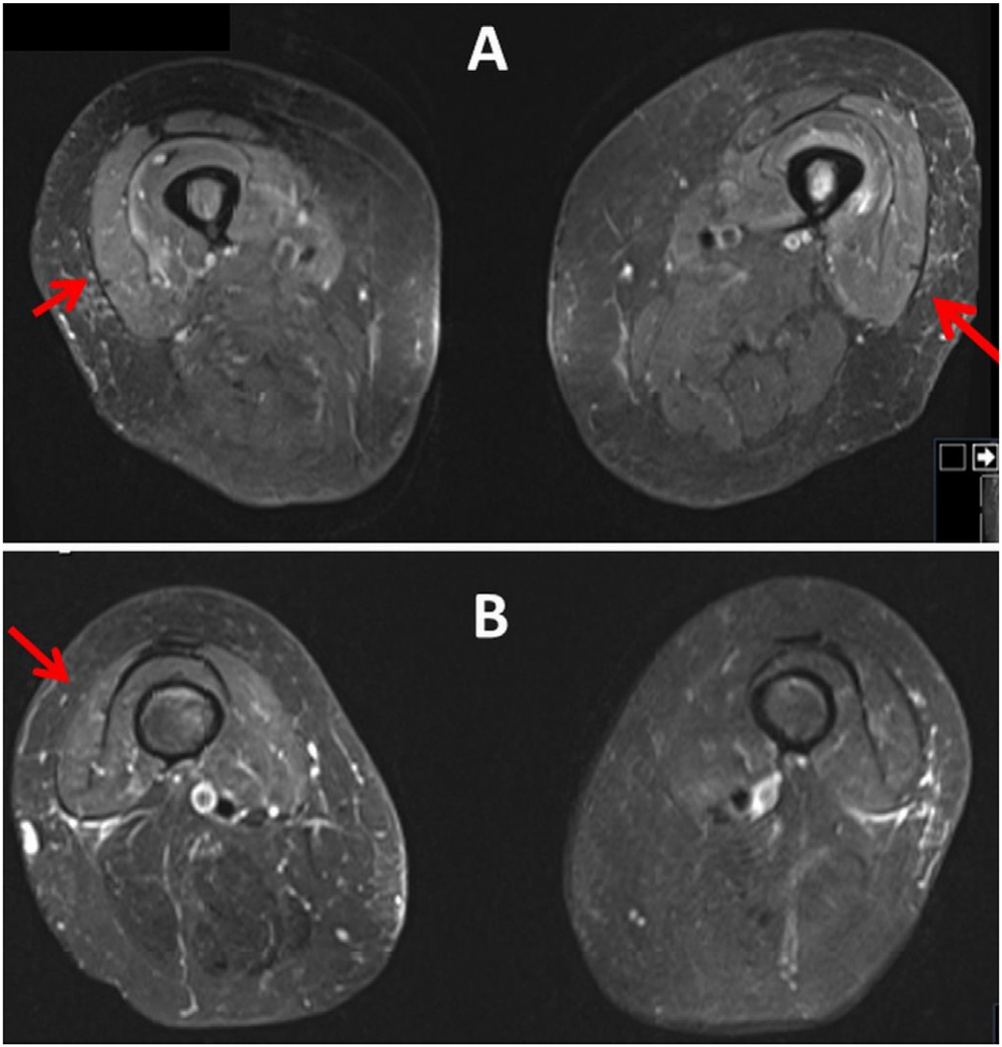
Muscle MRI (T2 STIR sequences) of the thigh and pelvic muscles three days after admission showing edema of the left intermedial vastus muscle, of the right medial vastus muscle, and right medial gluteus muscle arrows).
